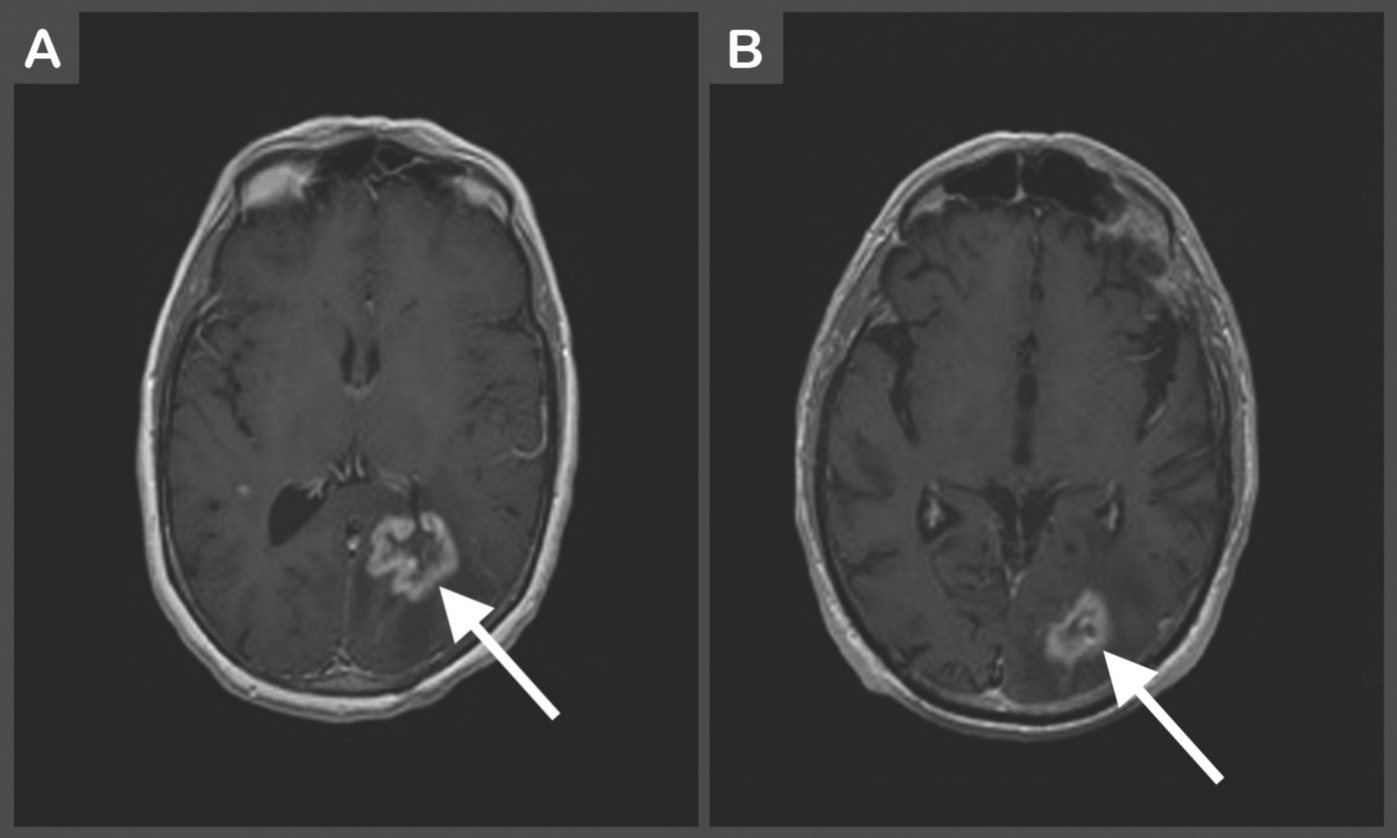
(A) Magnetic resonance contrast-enhanced T1-weighted image of left parietal lobe ring-enhancing lesion consistent with likely recurrent metastatic disease. (B) Magnetic resonance contrast-enhanced T1-weighted image showing enhancing lesion in the left occipital lobe suspected to be radiation-induced necrosis. This patient had undergone stereotactic radiosurgery with Gamma Knife two years prior. Gamma Knife: Elekta, Stockholm, Sweden
